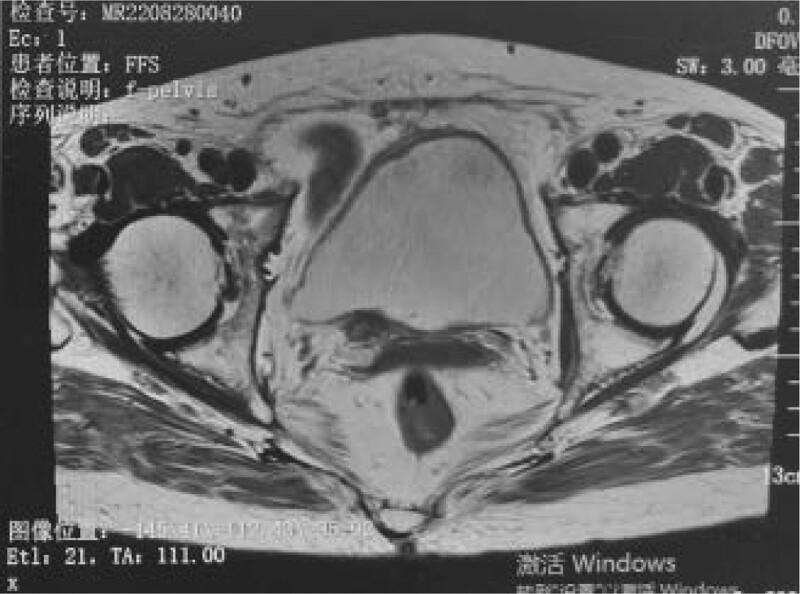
Postoperative MRI review. Limited thickening of the bladder wall at the entrance of the right ureter, low T2-weighted signal in the lesion area, and the possibility of recurrence. MRI = magnetic resonance imaging.
